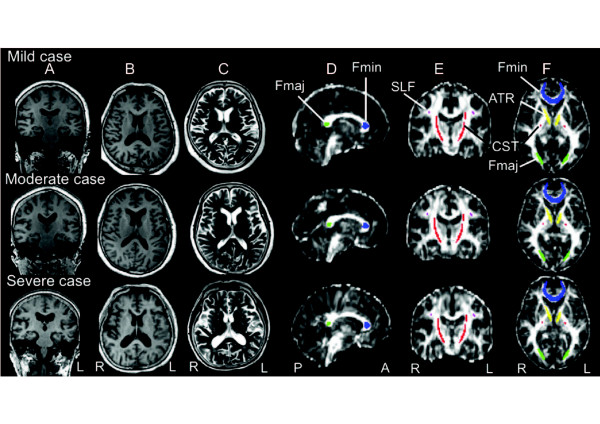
Magnetic resonance images from three Parkinson’s disease (PD) patients in arranged descending order of anterior thalamic radiation fractional anisotropy (FA) level (mild, moderate and severe). A) T1-weighted image (coronal view). B) T1-weighted image (axial view). C) T2-weighted image (axial view). D) Diffusion tensor image FA map (sagittal view). E) Diffusion tensor image FA map (coronal view). F) Diffusion tensor image FA map (axial view). Regions of interest (ROI) colors as for Figure 1. FA maps (FA values) of patients with PD (Figure 2D, E, F) show higher intensity (higher FA values) in white matter than those of patients with INPH (Figure 1 D, E, F).
